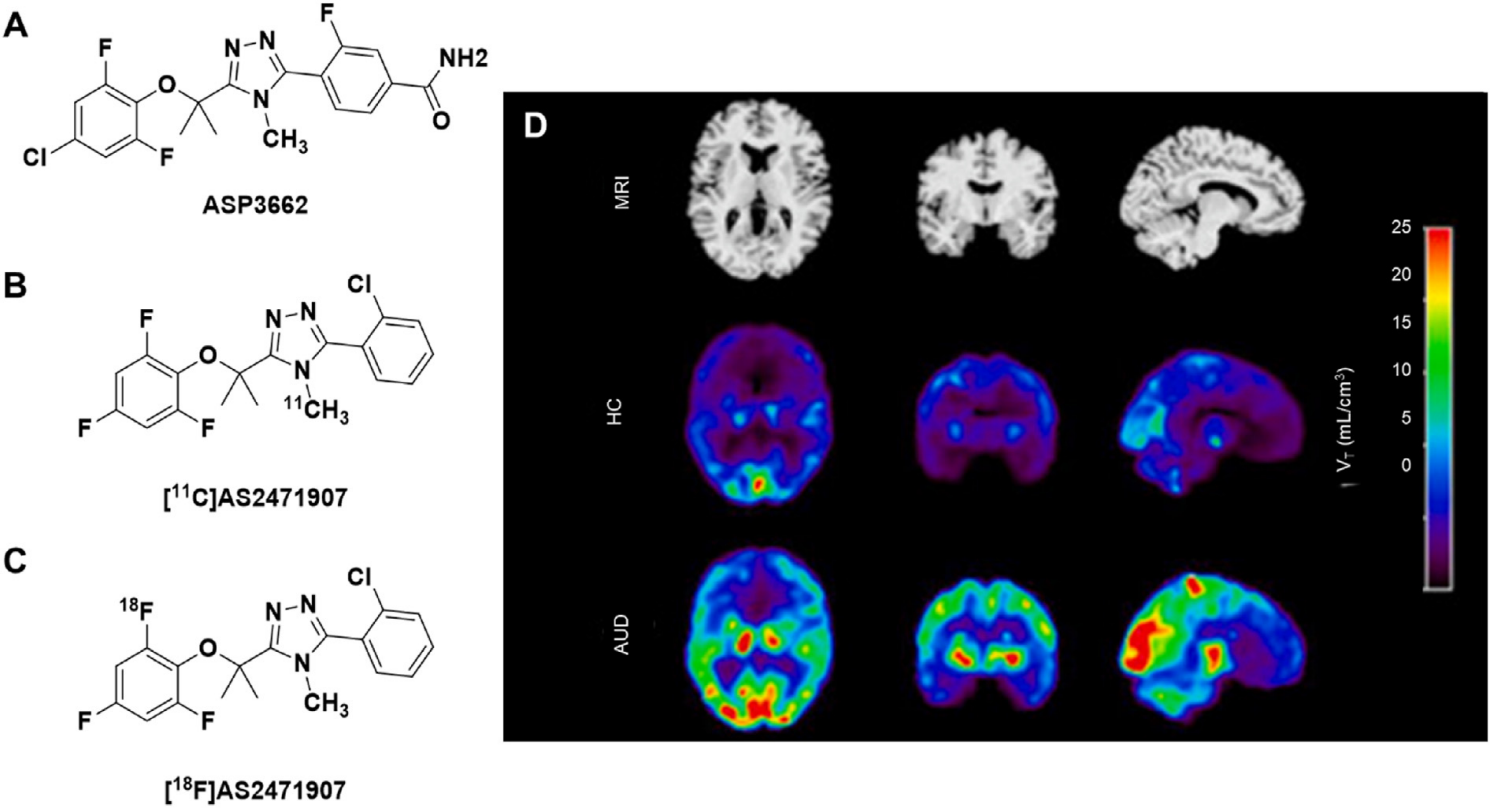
Structures of 11β-HSD1 radiotracers (A) [^11^C]AS2471907 and (B) [^18^F]AS2471907. (C) Participant's MRI and co-registered parametric [^18^F]AS2471907 *V*_T_ images in age-matched and sex-matched individuals with alcohol-use disorder (AUD) versus healthy control (HC). Adapted from Verplaetse TL et al. Imaging a putative marker of brain cortisol regulation in alcohol use disorder. Neurobiol Stress 2024; 29:100609. Copyright (2024), with permission from Elsevier.
